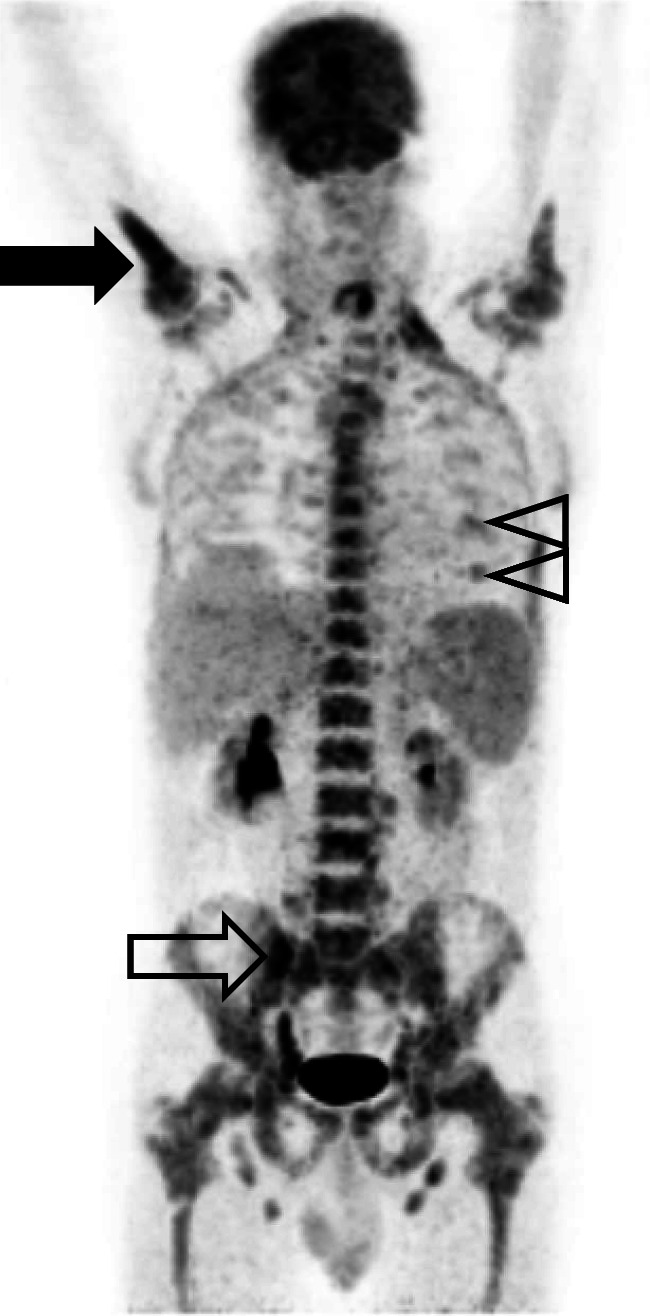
Baseline FDG PET (maximumintensity projection in frontal view) of a male patient with diffuse large B-cell lymphoma. FDG uptake in bone marrow space (with an intensity markedly above that in the liver) is diffusely increased without any focus in the bone marrow space apart from the biopsy site (right iliac crest, outlined arrow), but with asymmetric uptake (filled arrow) in right and left proximal humerus (maximum standardized uptake value 7.0 and 3.6, respectively) consistent with bone marrow involvement as confirmed by bone marrow biopsy. CT (not shown) revealed no osseous involvement in the proximal right humerus. Focal uptake in cartilage-bone junctions (outlined arrow-heads) as foci attributable to benign conditions
